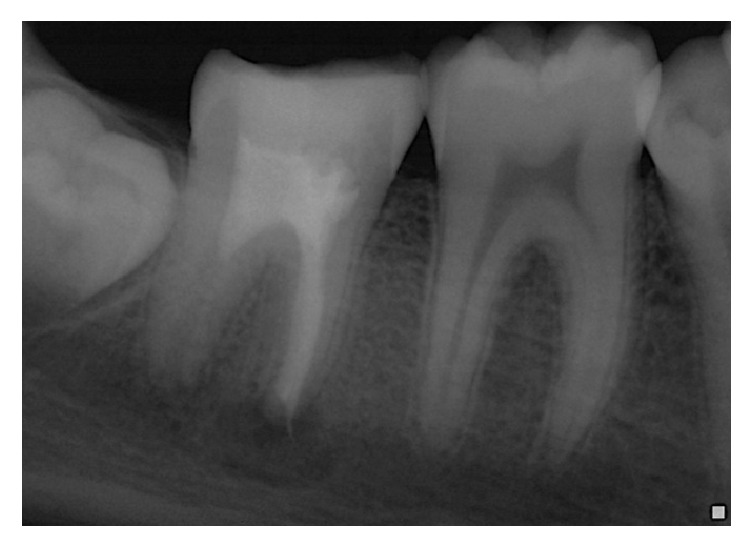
After 3 months from endodontic procedure on mesial roots: reduction of the radiolucent image at the mesial roots; healing of the periapical area at the distal root.
